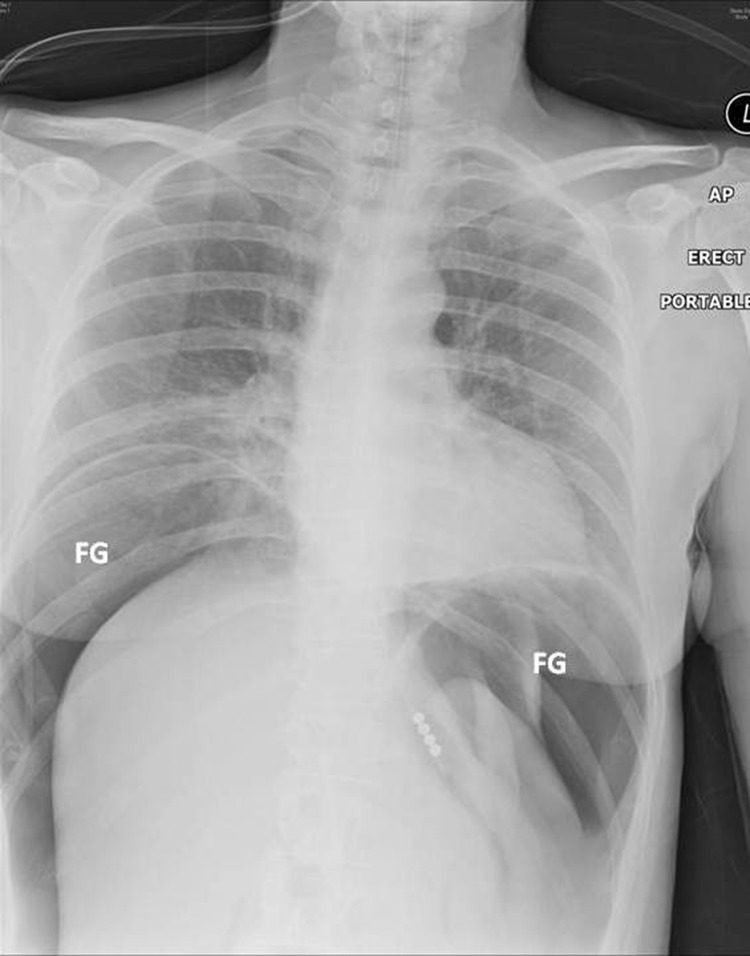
Chest x-ray showing free air under the diaphragm but no evidence of pneumomediastinum
